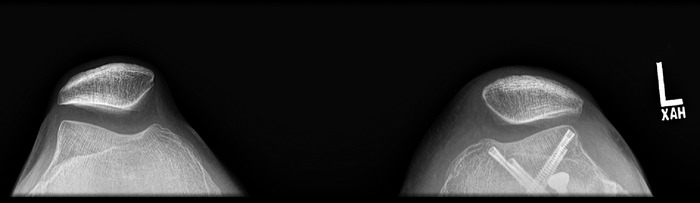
Merchant view radiograph of the left knee shows postoperative alignment following trochleoplasty.
